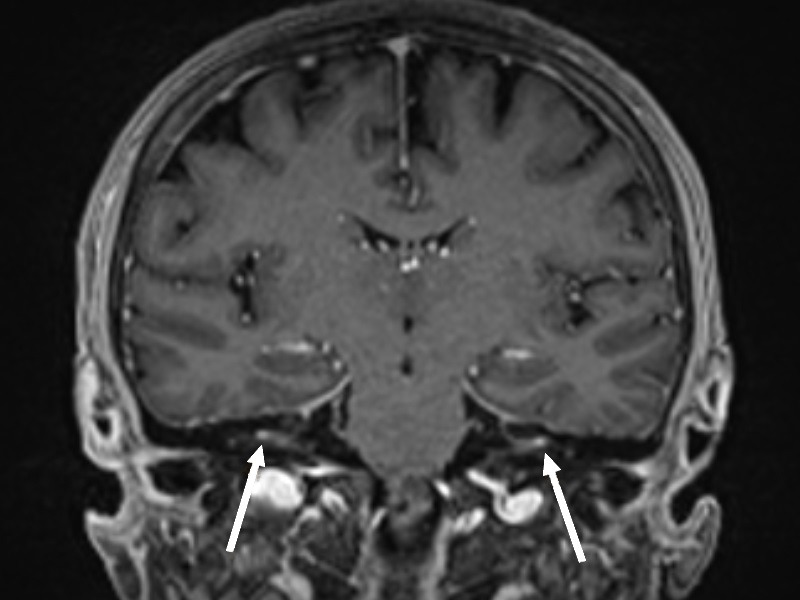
Magnetic resonance imaging (1.5T) findings: a coronal contrast-enhanced thin section (after i.v. gadolinium application). The T1-weighted image shows bilateral intrameatal facial nerve enhancement (arrows).
